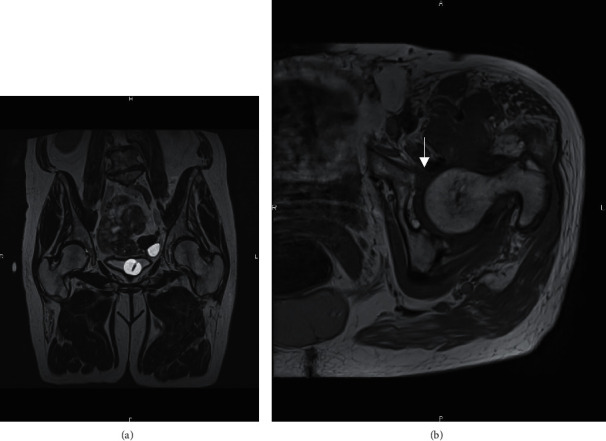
Pelvic MRI showing a focal anterior labral tear (arrow) with no evidence of soft tissue interposition. (a) Coronal view. (b) Axial view.
